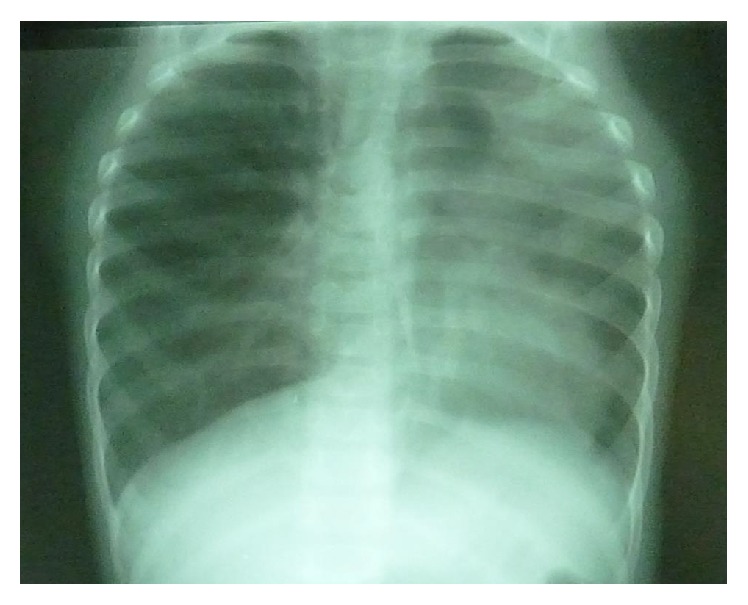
Chest X-ray showing left upper lobe collapse and mediastinal shift to the ipsilateral side.
